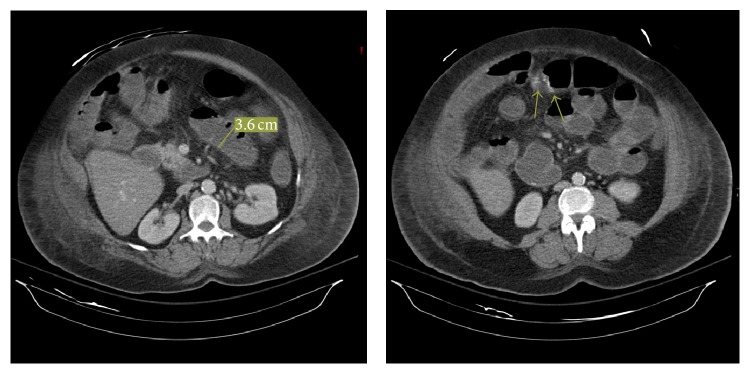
Computed tomography of the abdomen and pelvis with IV and PO contrast demonstrated diffuse dilation and fluid filling in the small bowel to the level of the ileocolic anastomosis out of proportion to the large bowel gas concerning for low-grade small bowel obstruction versus postoperative ileus.
